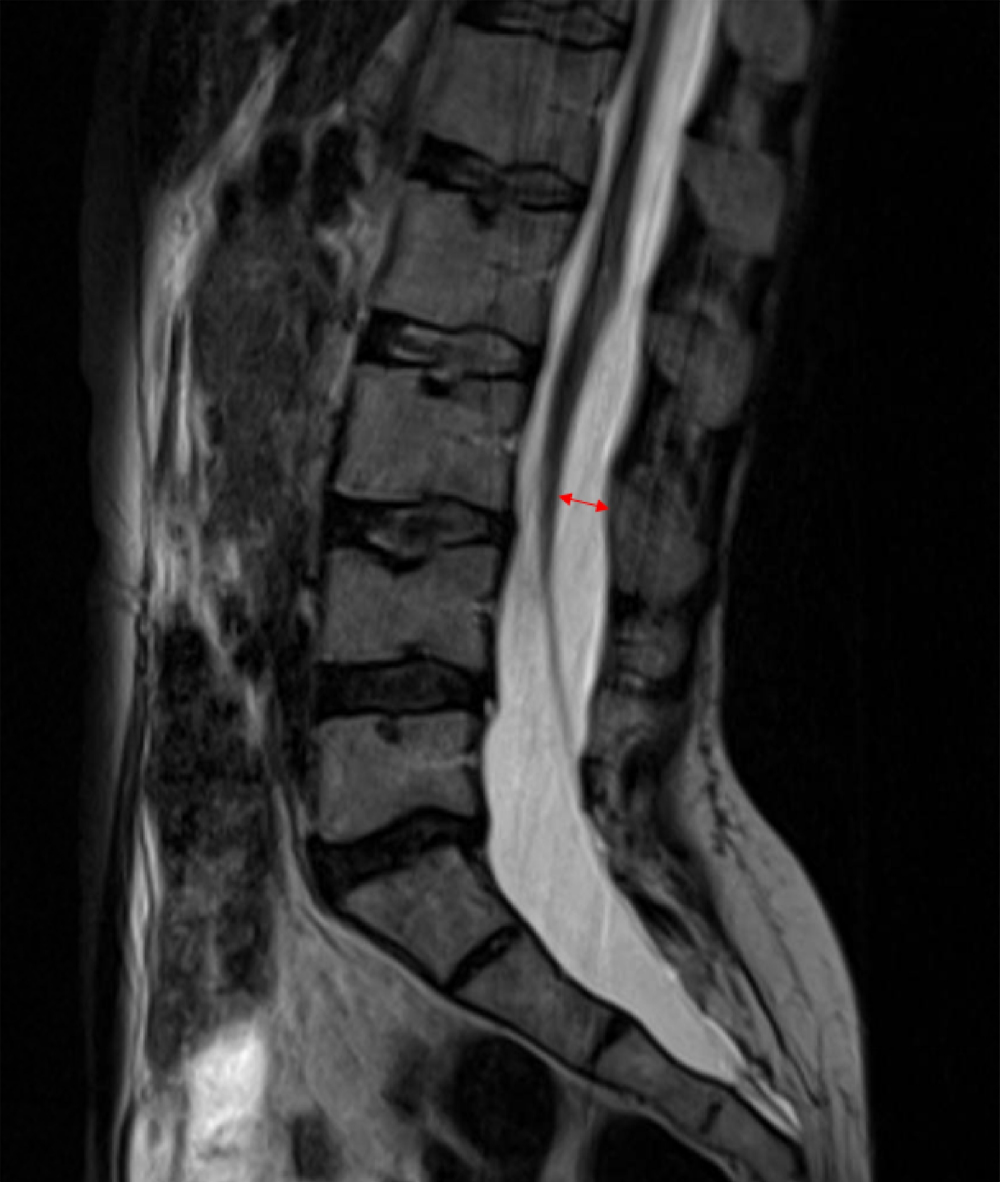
Sagittal T2 magnetic resonance imaging scan in the prone position showing adequate anterior movement of the conus compared to the supine imaging (red double arrow)
